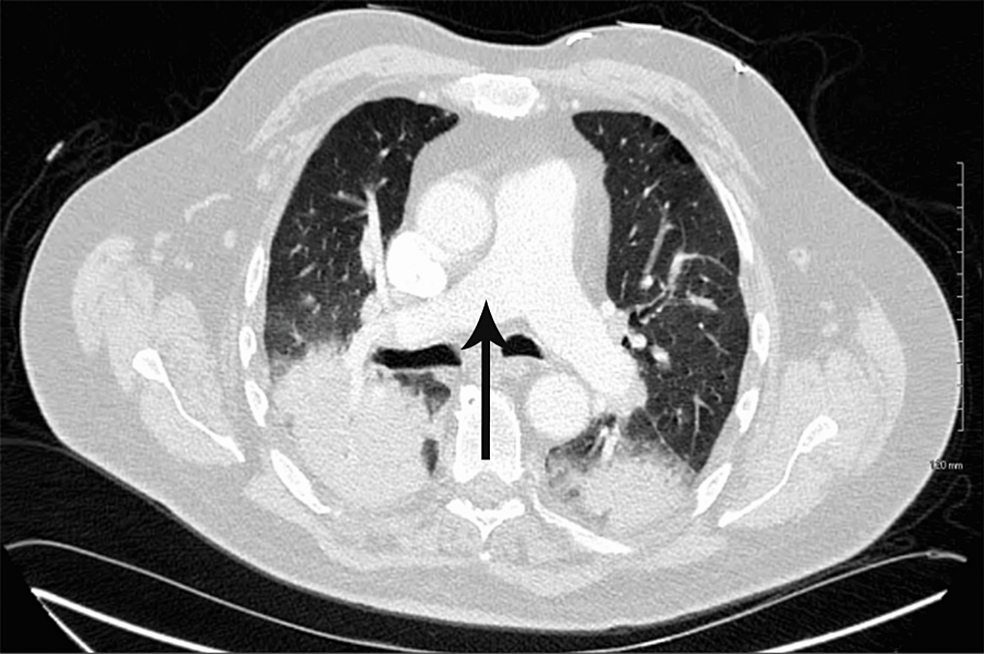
CT scan of the chest with contrast showing no evidence of pulmonary embolism (arrow) CT: computed tomography
